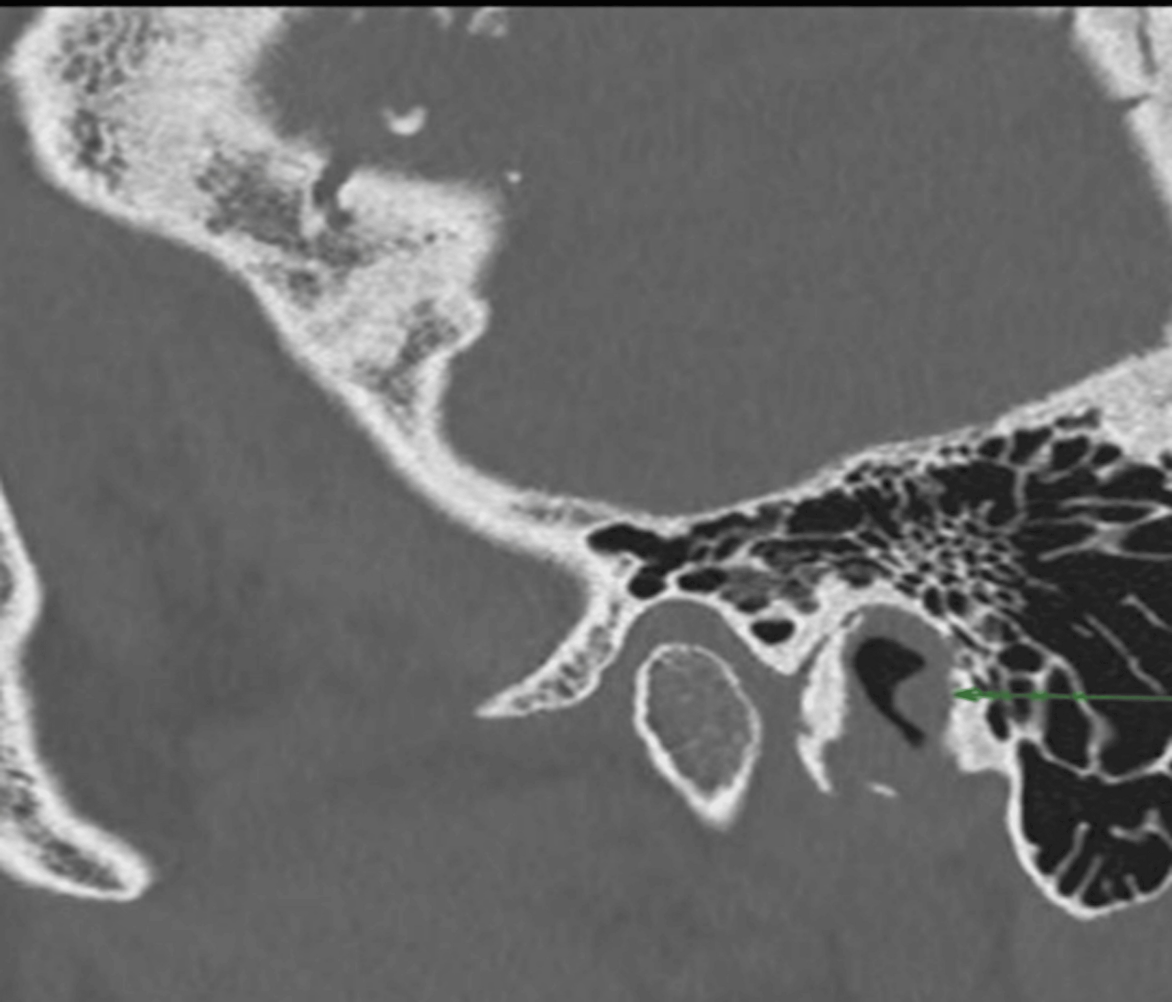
CT scan (sagittal view) of the left external auditory canal showing a 6 × 6 mm soft tissue lesion without bony erosion.
